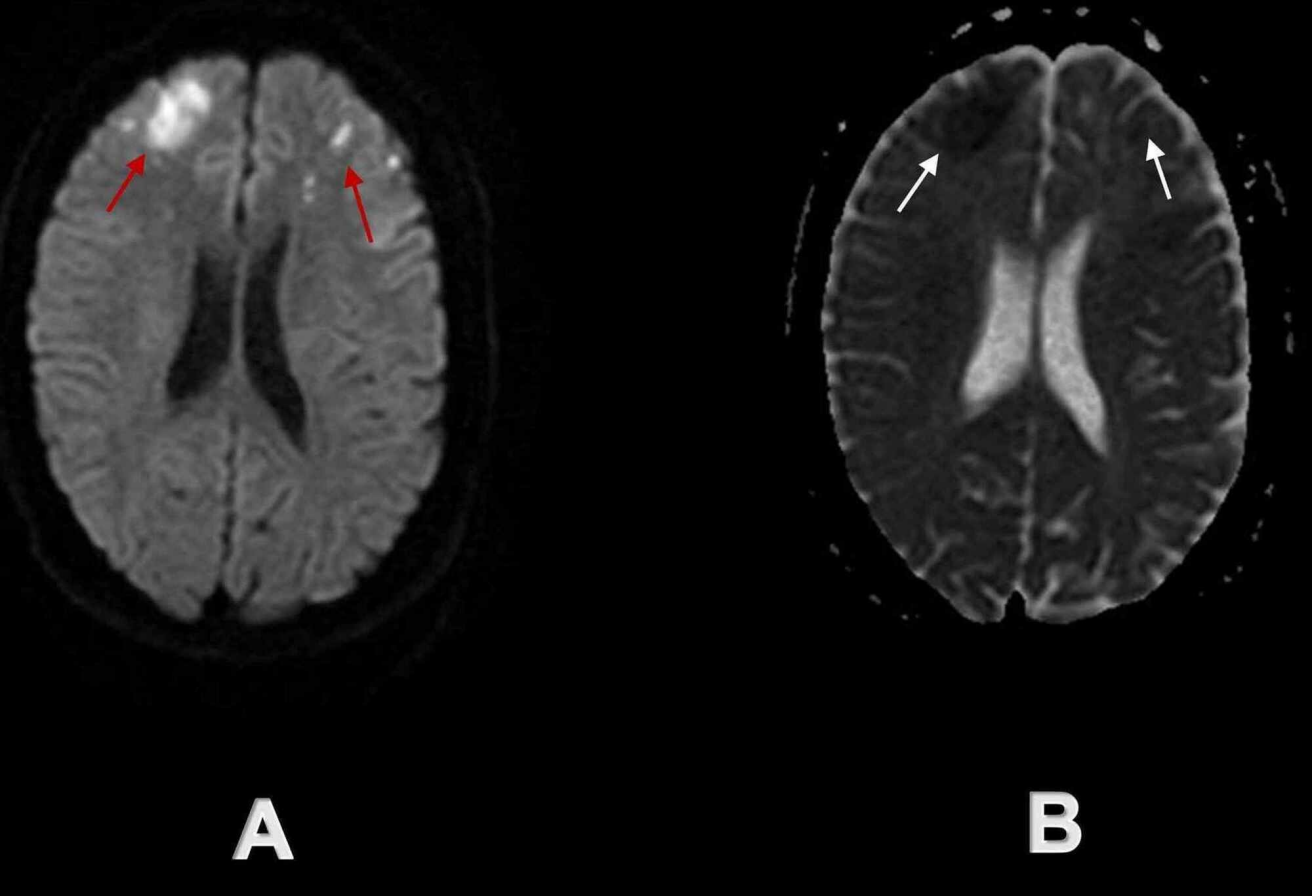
Initial brain MRI demonstrated multiple acute ischemic infarcts in bilateral frontal lobes Red arrows on axial DW sequence (A) and white arrows on axial ADC sequence (B) demonstrate multiple acute ischemic infarcts in bilateral frontal lobes. DW - diffusion-weighted; ADC - apparent-diffusion-coefficient
